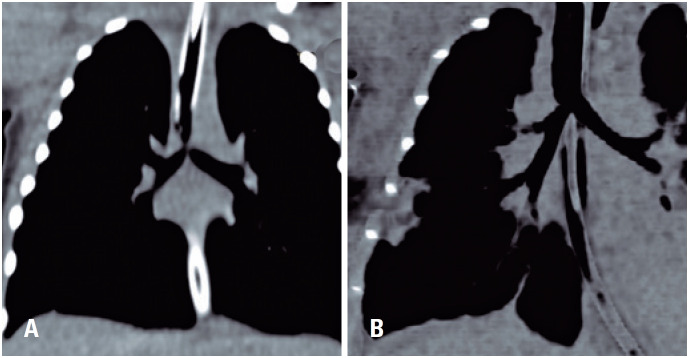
(A) Coronal reconstruction of the pre-operative chest CT with the stenosis in the distal trachea and carina; (B) Post-operative chest CT with adequate tracheal diameter
